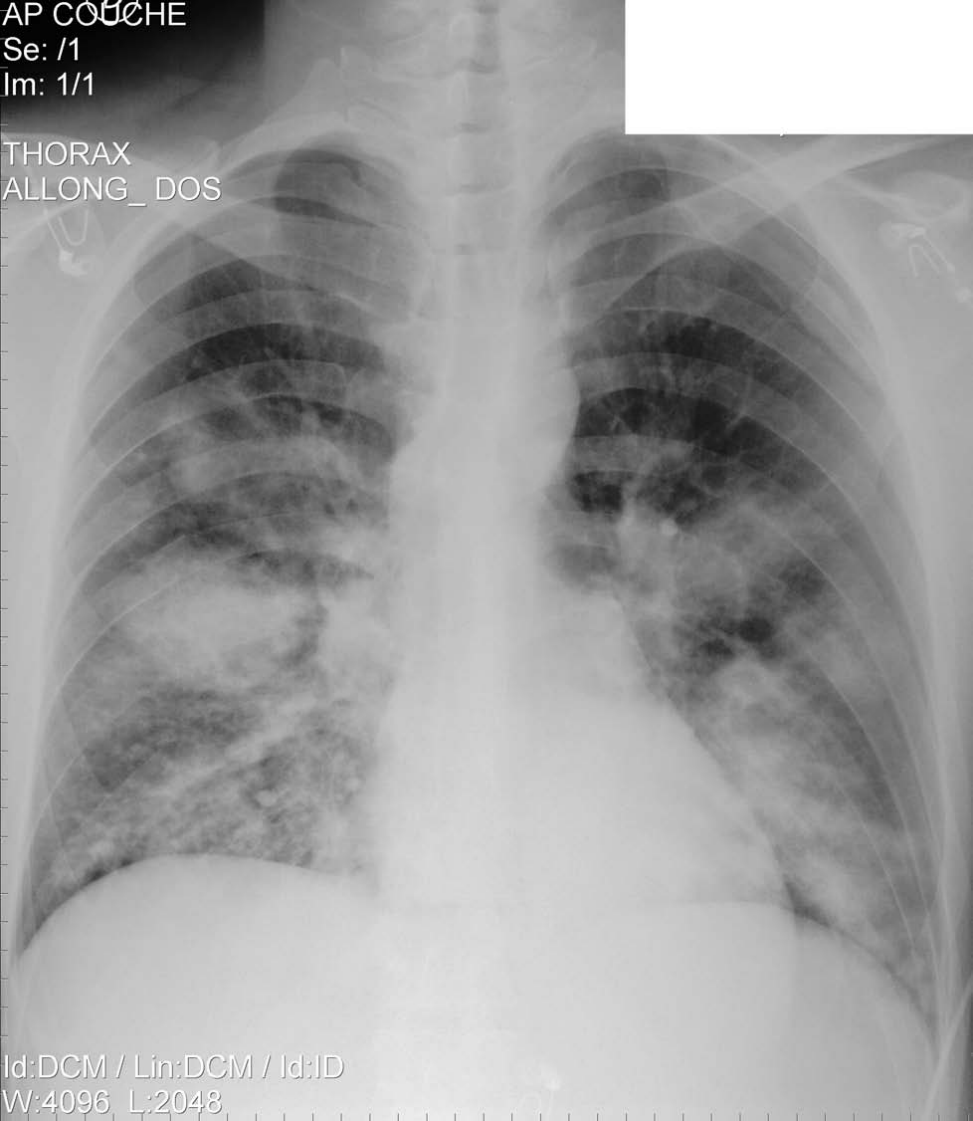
Chest radiograph on admission showing bilateral dense infiltrates.
